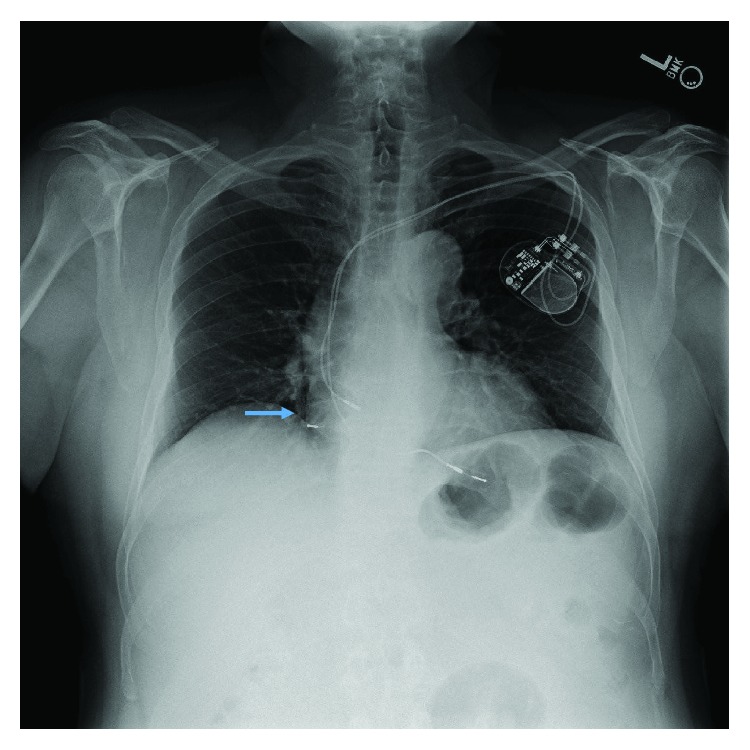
PA chest X-ray showed fractured right atrial lead (blue arrow).
